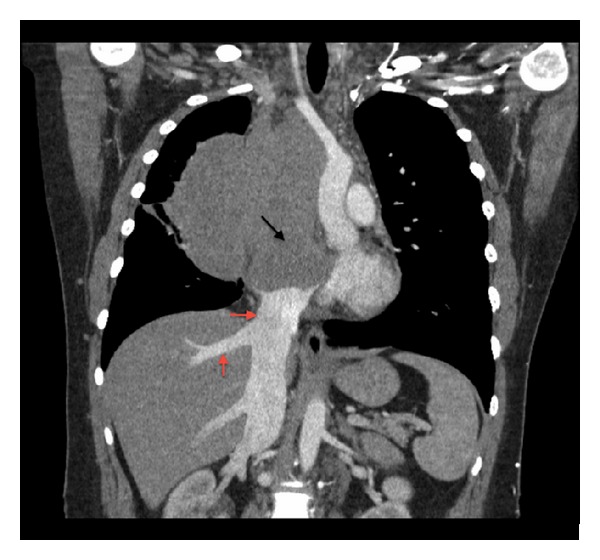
Coronal CT scan image elucidates a mediastinal mass with extension into the right atrium (black arrow) with complete encasement and compression of the SVC. The tumor extends to the confluence of the IVC in the right atrium causing dilatation of the intraabdominal IVC and hepatic veins suggesting compromised cardiac return (red arrows). Tumor causes the displacement of great vessels into the left hemithorax.
